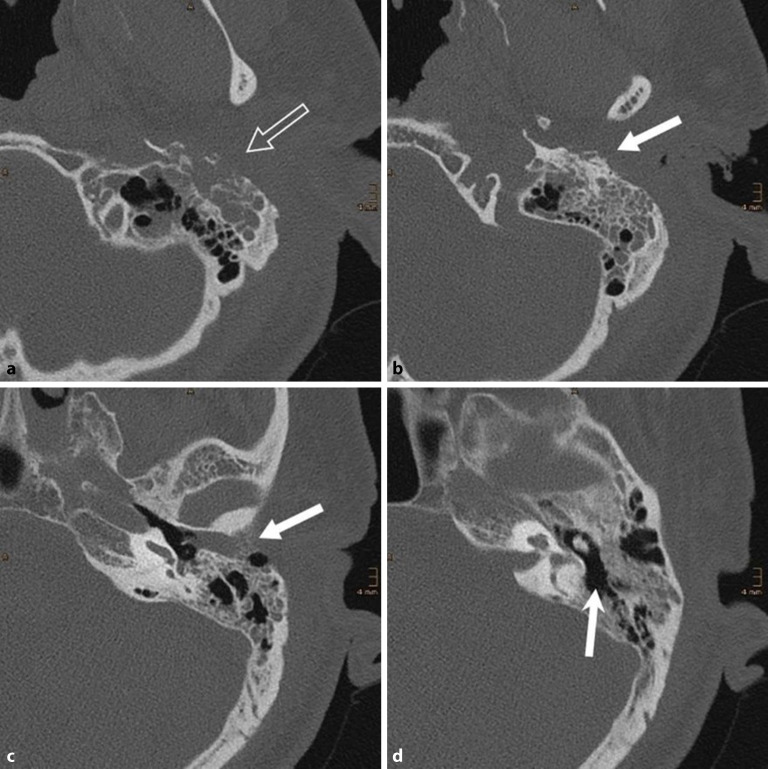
Felsenbein-Computertomographie (CT) bei Otitis externa necroticans bei einem 80-jährigen Mann mit a nekrotischer Zerstörung des vorderen Mastoids (*offener, nicht ausgefüllter Pfeil*), b,c entzündlicher Verlegung des äußeren Gehörgangs und nekrotischer Arrosion des unteren Randes (*weißer Pfeil*) sowie d luftgefülltem Paukenraum und Antrum (*weißer Pfeil mit offener Spitze*)
